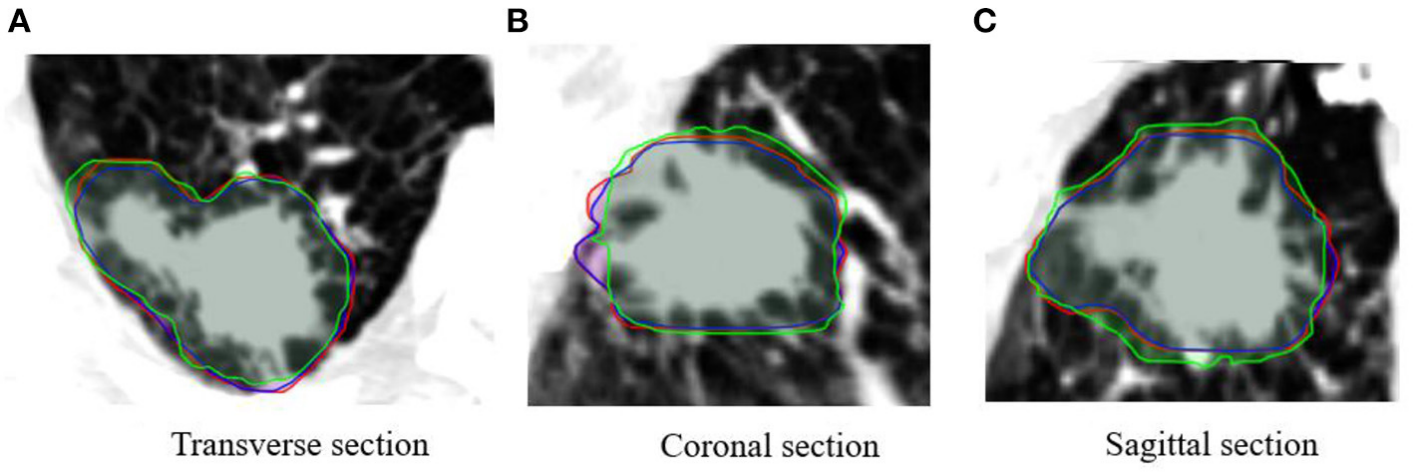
Comparison of contours between planning target volume on four-dimensional CT (PTV_4DCT_) (red), PTV_predict_ (blue), and PTV_FBCT_ (green) in different views of patient images (projected PTV_predict_ and PTV_FBCT_ on 4DCT images).
